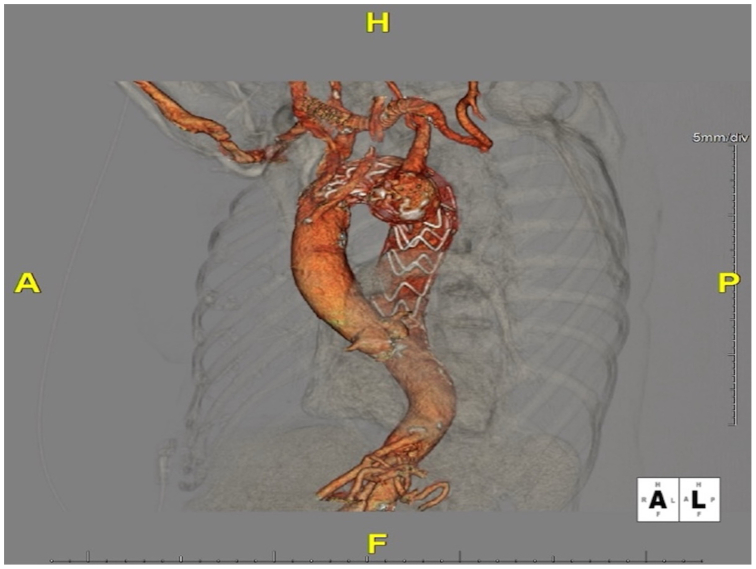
Postoperative three-dimensional computed tomography angiography (CTA) reconstruction after thoracic endovascular aortic repair (TEVAR) and coil embolization, showing successful placement of the thoracic endograft.
